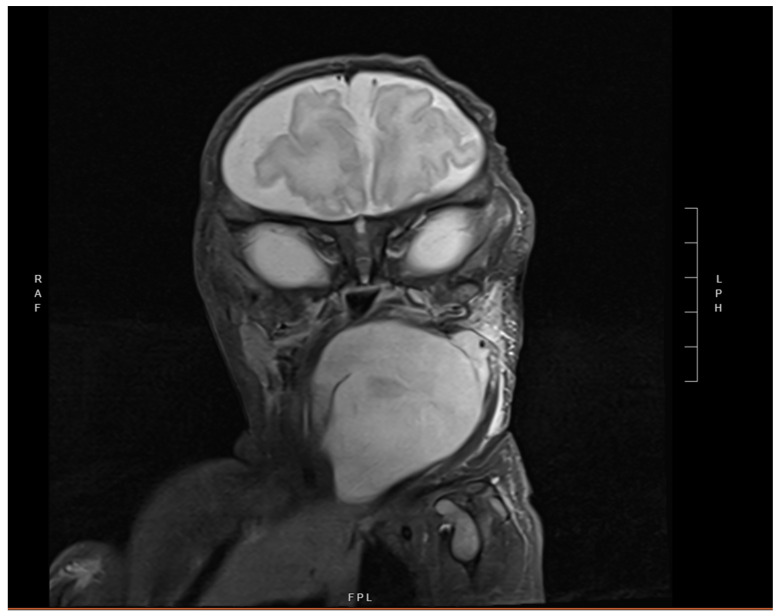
Coronal short tau inversion recovery (STIR) MRI image demonstrating 2.2 × 5.2 × 5.1 cm neck mass in left parapharyngeal space.
